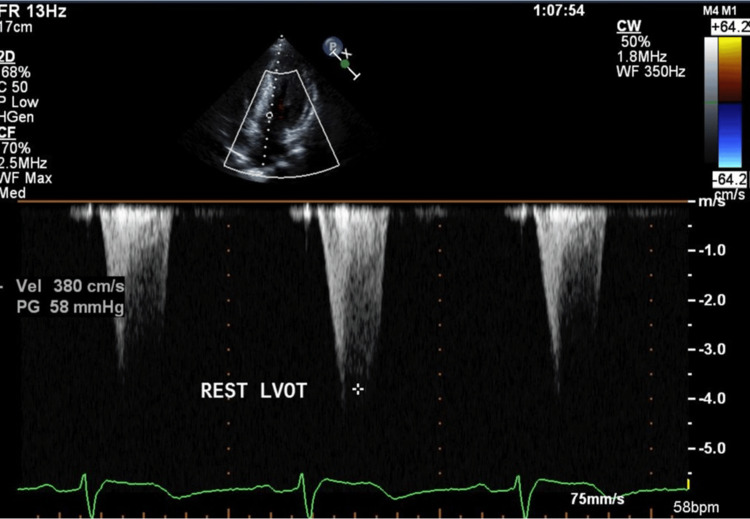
Echocardiography during the second pregnancy The left ventricular outflow tract pressure gradient was as high as 58 mmHg.
